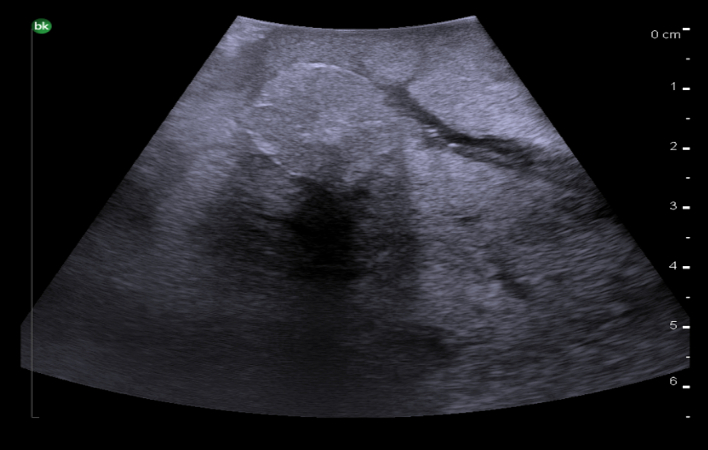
Intraoperative ultrasound appearance of an isoechoic hepatocellular carcinoma with a hypoechoic rim.
